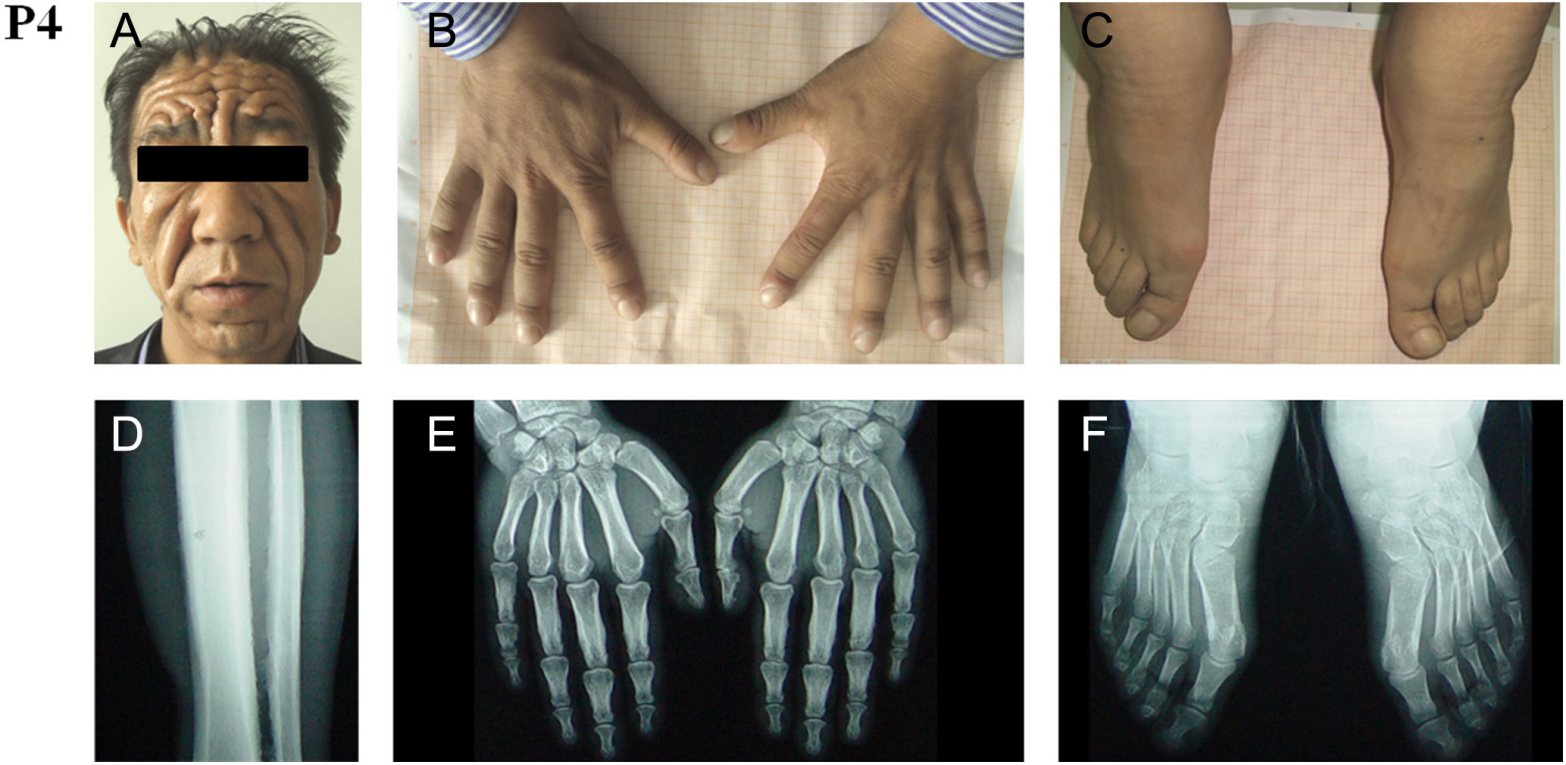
Clinical images of the affected individual: Family3, II.1-P4. The images showed the thickening and furrowing of facial skin (A) and the clubbing of fingernails and toenails (B and C). A radiograph of tibiofibula showed periosteal hyperostosis (D). A radiograph of the hands and feet showed cortical thickening and acroosteolysis (E and F). All images were published with permission from the affected individual.
